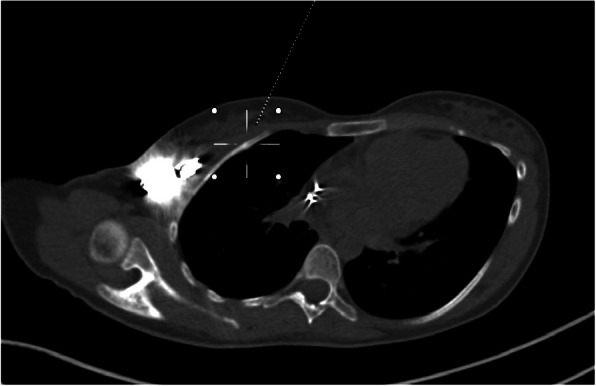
Computed tomography (CT) image in oblique axial plane depicting pacemaker generator and a healing fracture (dotted line) in the anterolateral part of the second right rib
